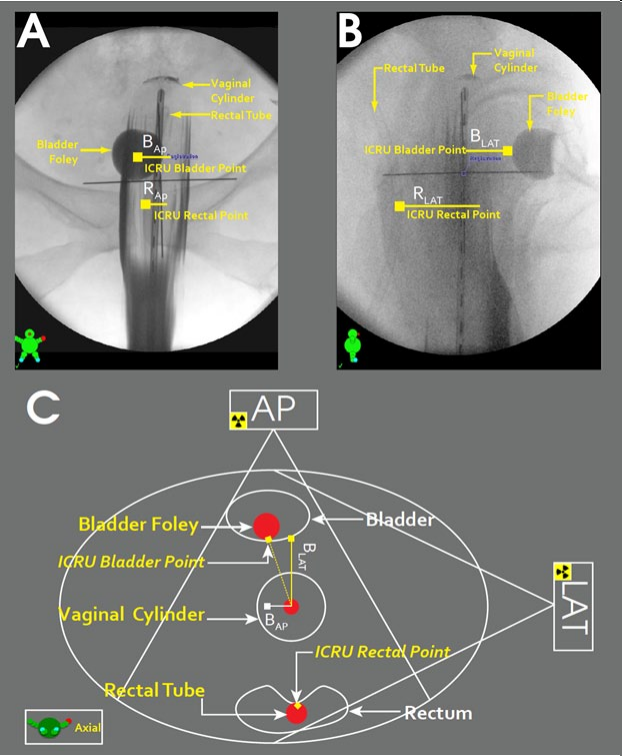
Anterior–posterior (AP) (a) and lateral (LAT) (b) view of an orthogonal radiograph set. Conceptualization of the corresponding axial view (c).
